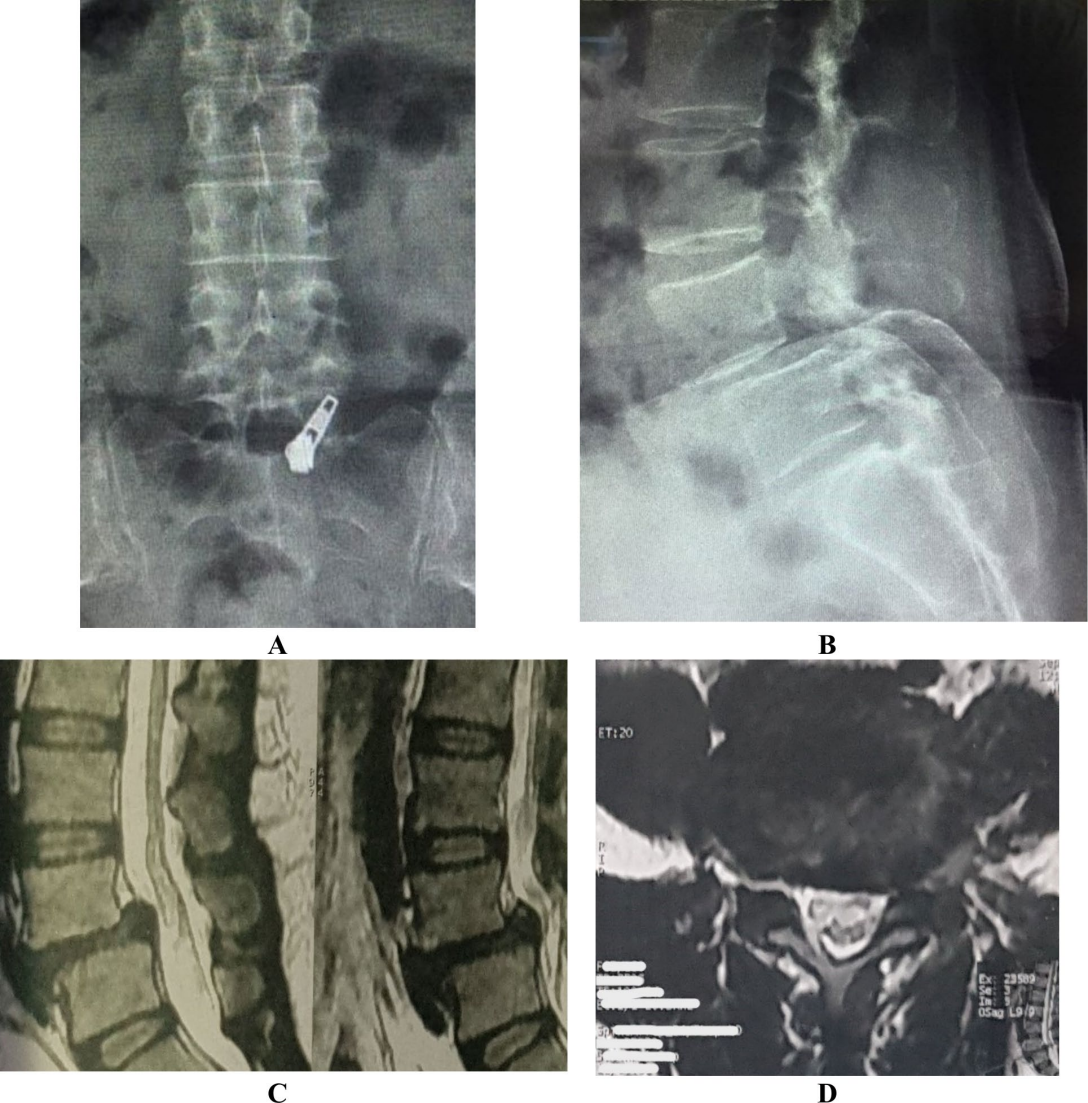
MISTLIF case: (A-B) show antero-posterior and lateral radiology of the slipped level and (C-D) show the MRI photo of that level (sagittal and axial cut)
